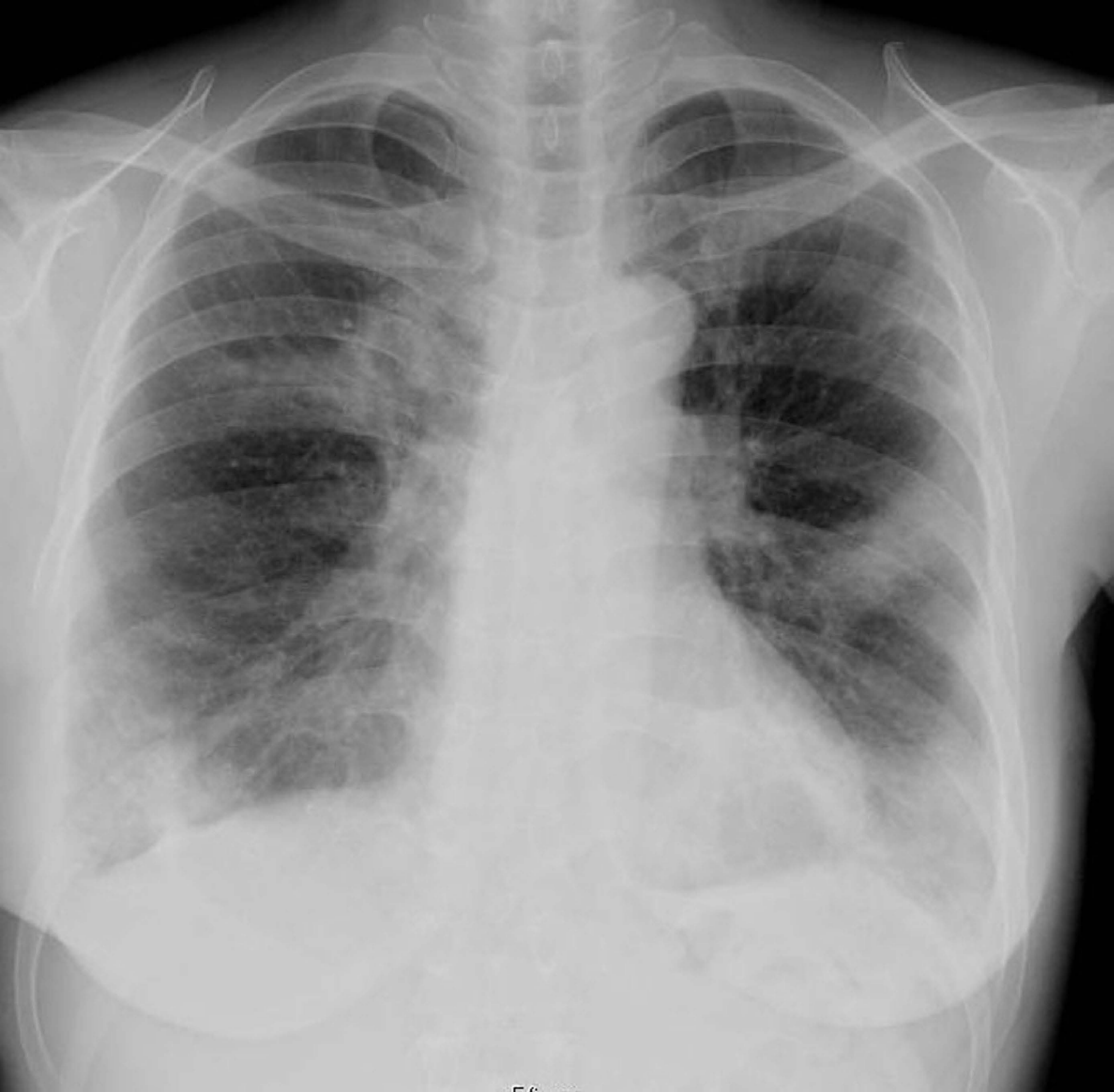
Chest X-ray*. *Chest roentgenogram showed air-space consolidation with an air bronchogram, predominantly in the right upper and left lower lung fields*.
